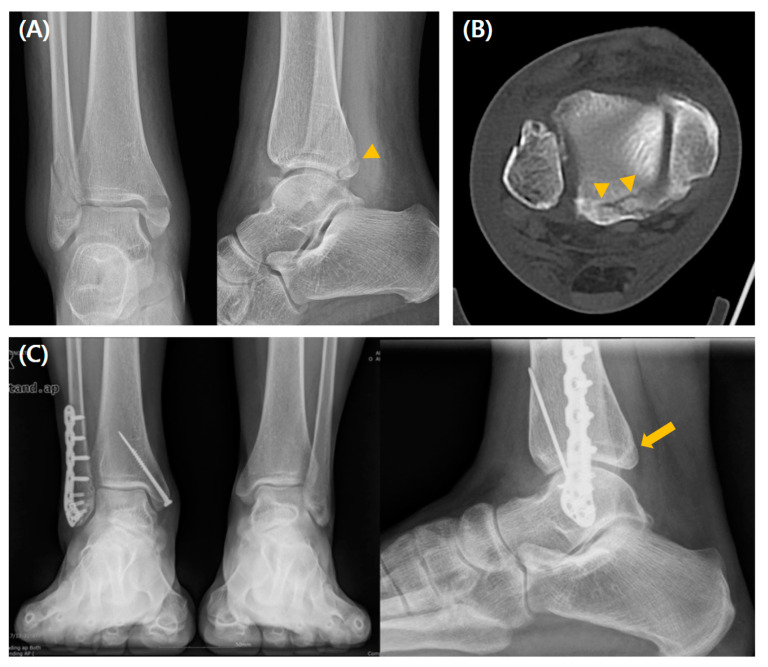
A case of a 34-year-old man who underwent conservative management without syndesmotic stabilization for type 3 PMF. (A) Preoperative radiographs show a small avulsion-type posterior lip fracture (arrowhead), and (B) preoperative CT scan images show a posterior malleolar fragment representing a type 3 fracture (arrowhead). (C) Weight-bearing radiographs obtained 2 years postoperatively show good reduction and bone healing (arrow).
